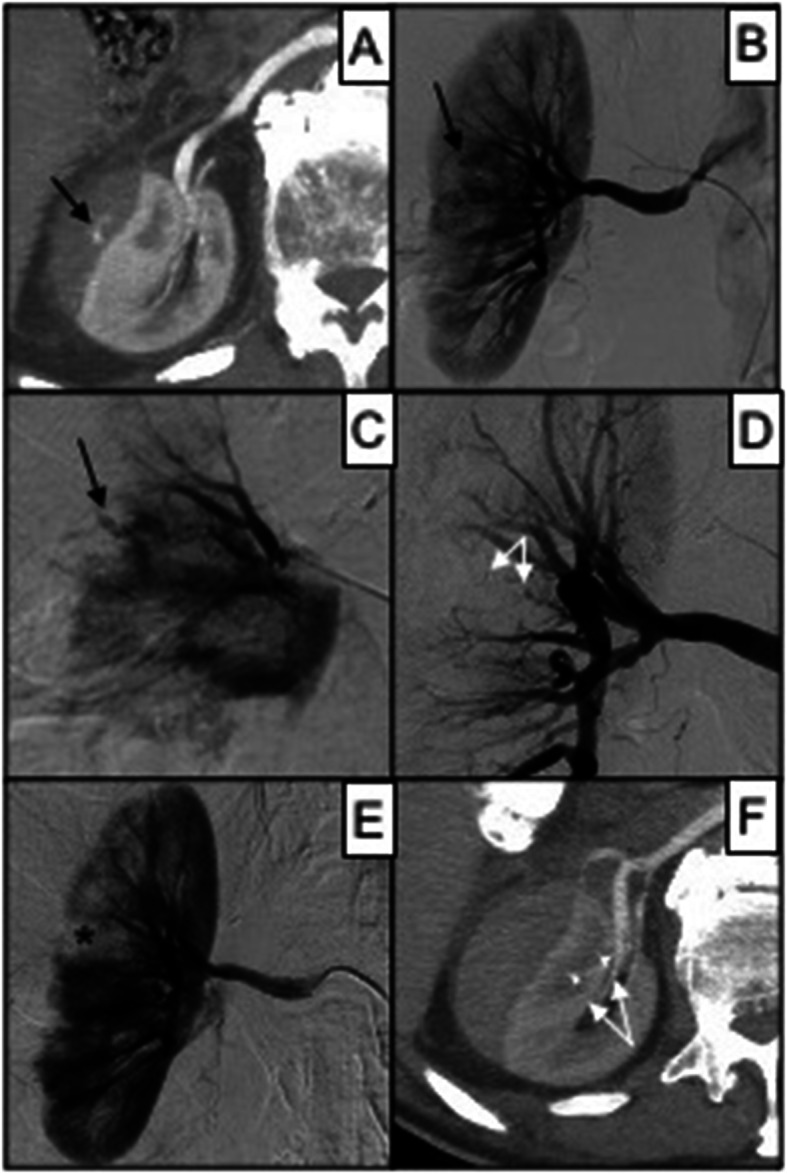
86 years old male affected by renal bleeding after car investment. In A, a contrast-enhanced CT scan in arterial phase shows perirenal hematoma of the right kidney refurnished by active bleeding (black arrow); in B, DSA confirms active bleeding (black arrow) from a distal intraparenchymal branch of the right renal artery; in C, superselective DSA shows the bleeding vessel (black arrow); in D, superselective DSA shows bleeding resolution after MVP-3 release (target vessel caliper 1.3 mm); in E, renal DSA confirms proper embolization with minimal ischemic area (black asterisk); in F, day after CT scan follow-up shows effective embolization without MVP (white arrows) metallic artifacts
